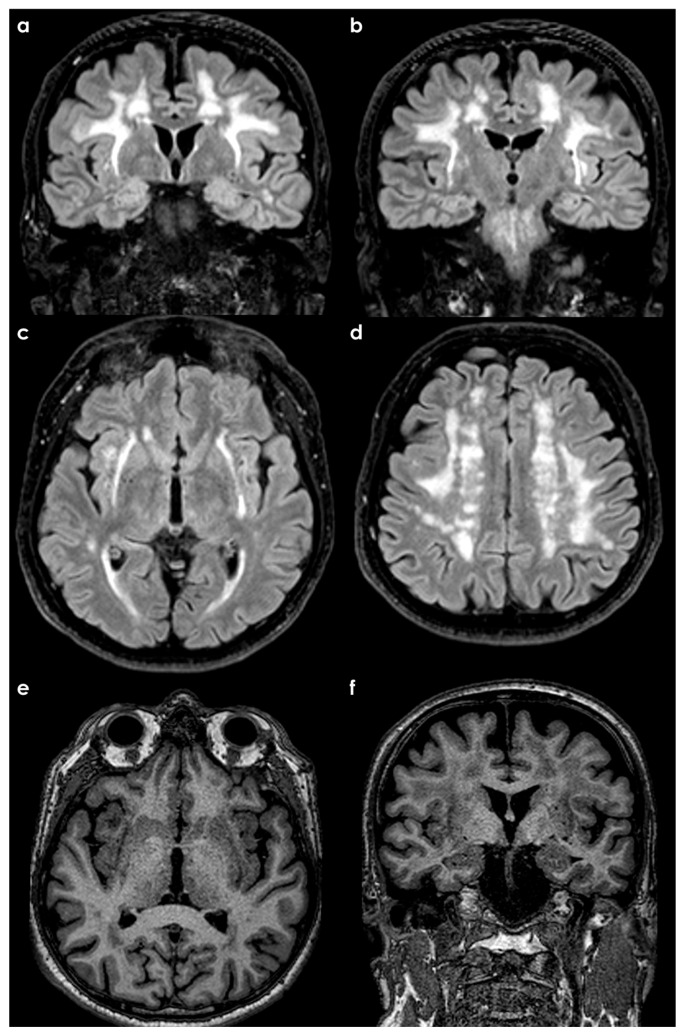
Brain MRI of a patient with extensive SVD and mild lower body parkinsonism with memory complaints. Panels (a–d) show FLAIR (coronal in (a,b), axial in (c,d)) sequences with extensive white matter hyperintensities extending along the external capsule on both sides and in the extreme capsula on the right side. Panels (e,f) show the corresponding axial and coronal T1W sequences with a hypointense and easily identifiable claustrum on both sides.
